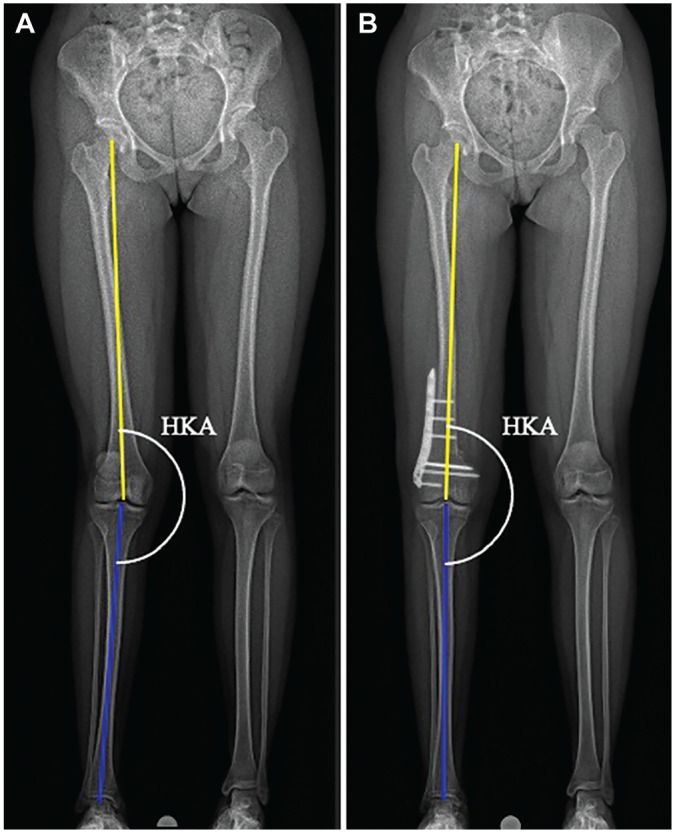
Weightbearing (A) preoperative and (B) postoperative long-leg radiographs showing measurement of the hip-knee-ankle angle (HKA) of the right leg. The top line determines the mechanical axis of the femur, and the bottom line the mechanical axis of the tibia.
